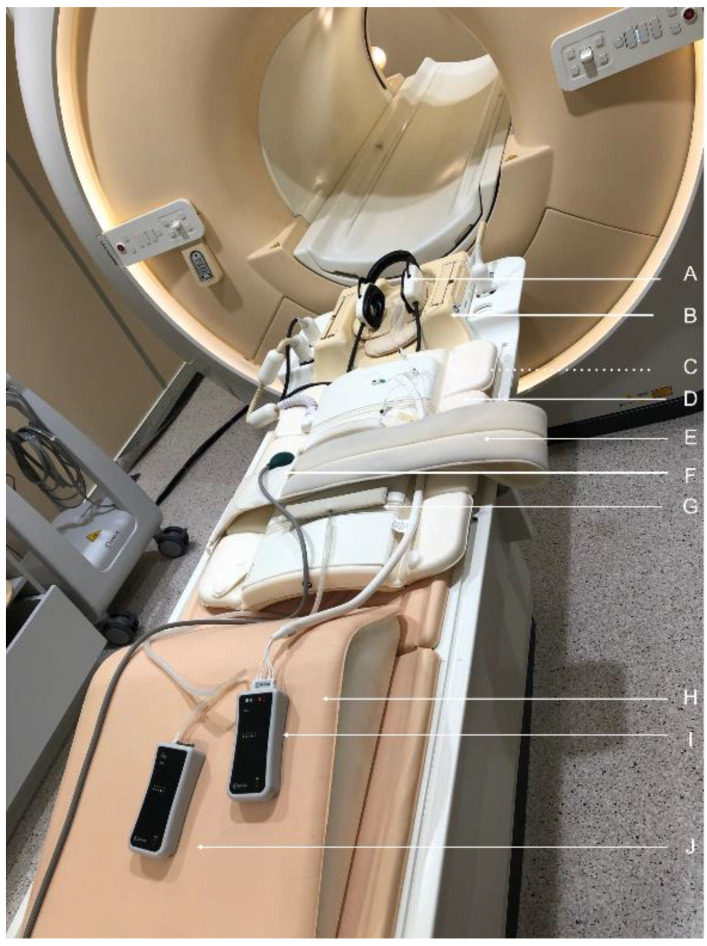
A set of MRI accessories usually used in CMR procedures, located on the MRI table following the usual order when a patient is prepared for an examination: (i) ear protection/headphones, EP, (A) located at the lower part of two-piece head coil, TP-C, and head stabiliser, HS, (B); (ii) cardiac monitor, CM, electrodes (C); (iii) body surface coil/flexible, BS-C, (D) and a stabilising strap (E); (iv) communication ball, CB, (F); (v) respiratory monitor, RM, sensor (G); (vi) lower limb stabiliser, LLS, (H); (vii) CM module (I); (viii) RM module (J).
